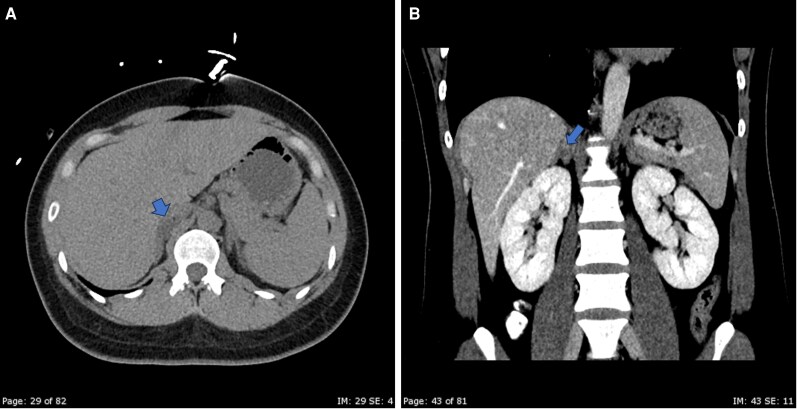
Axial (A) noncontrast and coronal (B) contrast-enhanced computed tomography (CT) images showing a well-defined, homogeneous right adrenal nodule (arrows), measured 1.3 cm, with unenhanced attenuation of 15 Hounsfield units. The coronal image highlights the spatial relationship of the lesion to the adjacent kidney and vasculature.
